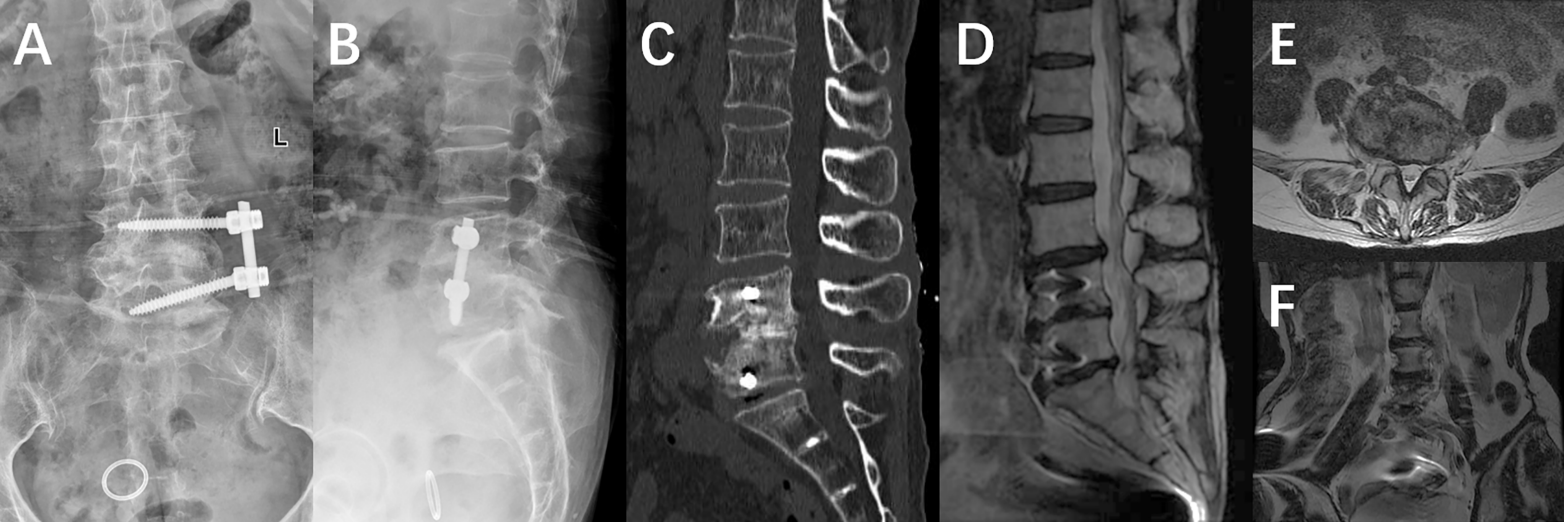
(A, B) An X-ray taken a week after the operation revealed that the internal fixation was in the correct position. (C–F) The postoperative MRI indicated that the abscess had been completely drained and cleared after the Negative Pressure Wound Therapy treatment.
